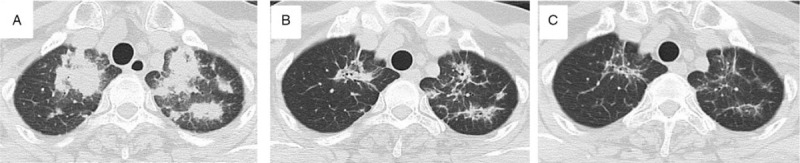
A, Chest CT show multiple patchy lesions appeared in the bilateral upper lungs. B, Treatment 2 weeks later. C, Treatment 1 month later. CT = computer tomography.
